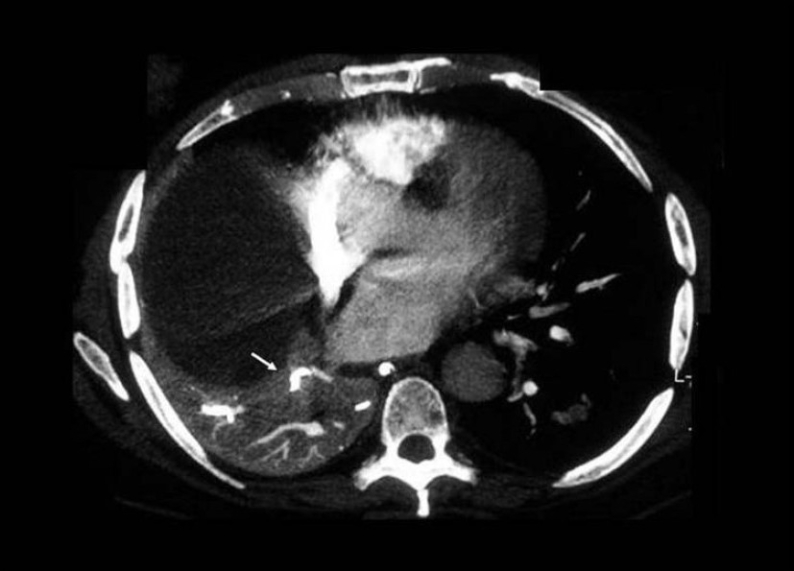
An axial CT of the same patient as in Figure 34 showing tubular opacities of metallic density due to acrylic cement pulmonary embolism as a complication of vertebroplasty
